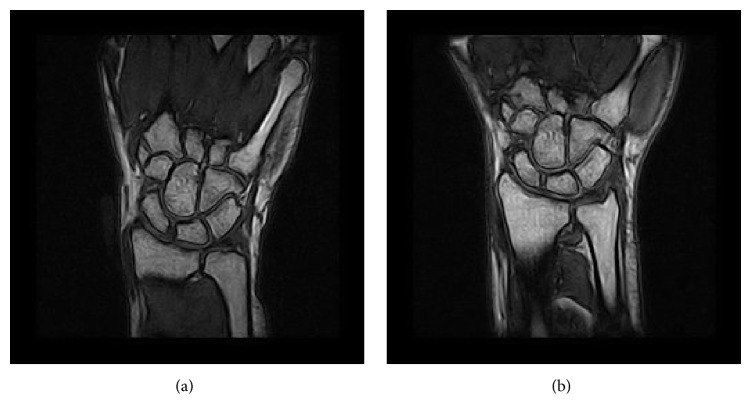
A sample coronal slice from the initial MRI spatial sequence (a) and the follow-up spatial sequence (b).
